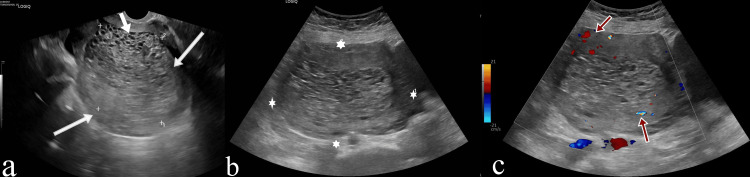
Grayscale transabdominal and transvaginal ultrasound images of the mass lesion (a) In the grayscale transvaginal ultrasound image, a mass lesion with a characteristic vesicular pattern is seen filling almost the entire endometrial cavity (white arrows). (b) In the grayscale transabdominal axial ultrasound image, an endometrial mass measuring approximately 13×9 cm is seen (asterisk). (c) In the axial plane color Doppler ultrasound image, the internal vascularity of the lesion is seen (red arrows).
